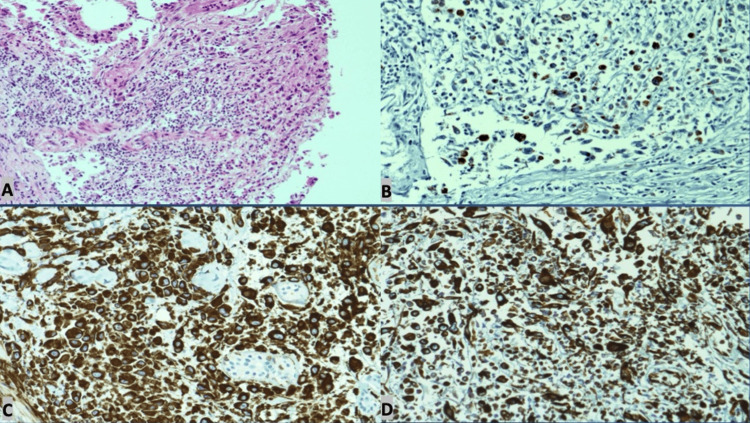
Renal biopsy at 10x magnification A: infiltrative poorly differentiated epithelioid to spindle cells exhibiting pleomorphic, hyperchromatic nuclei with prominent nucleoli and eosinophilic cytoplasm; B: tumor cells positive for EMA; C: tumor cells positive for panCK; D: tumor cells positive for vimentin CT: computed tomography; EMA: epithelial membrane antigen; panCK: pan-cytokeratin
